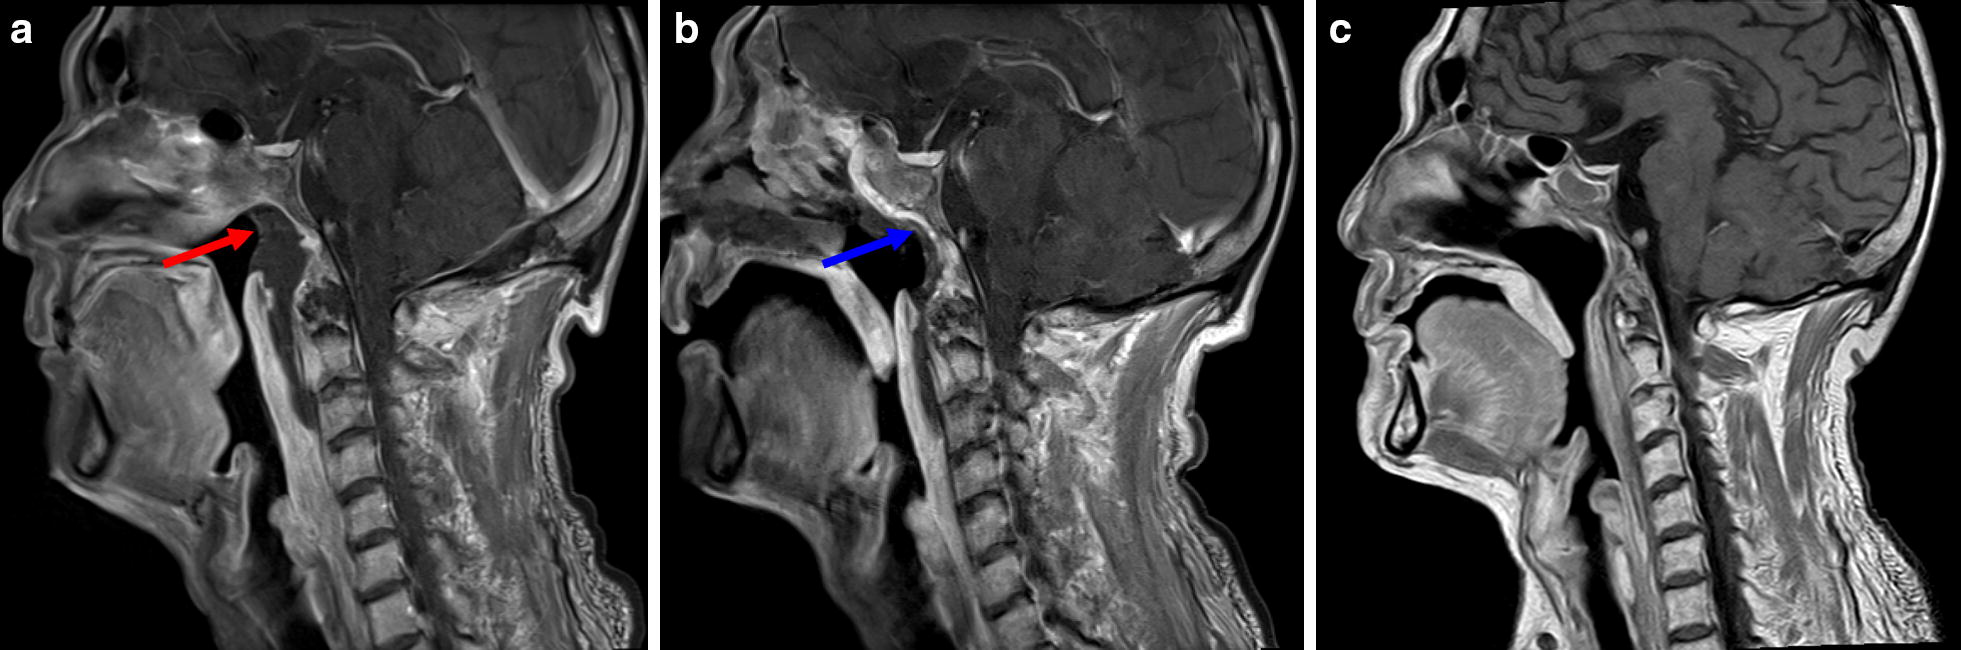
Contrast-enhanced sagittal magnetic resonance imaging (MRI) of the head and neck of a patient before and after surgery. a PRNN (indicated by the red arrow) was located in the roof of the posterior wall of the nasopharyngeal cavity and oral pharynx as shown on the preoperative MRI. b The flap (indicated by the blue arrow) re-surfaced the defect after radical endoscopic necrectomy, with good blood supply at 2 weeks after surgery. c The flap completely relined the nasopharyngeal mucosa at 8 weeks after surgery
